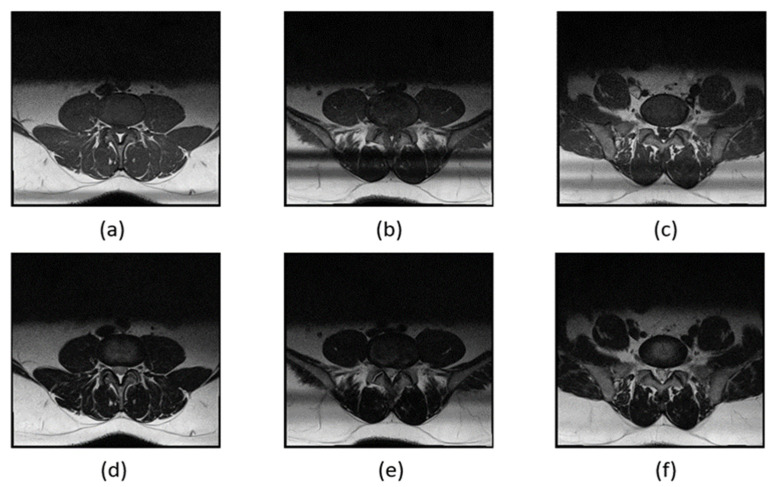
Spinal canal in different parts in T1–T2. From Figure 8, it can be observed that there are slight differences in pictures (a–f). (a) The facet joints of the spinal canal in the MRI images of L3 and L4 are more obvious, and the L5 site is connected to the S1 site; (b) therefore, the shape of the spinal canal in the MRI image will be more special. (c) T1 and T2 weighting also had a significant influence on the image. (d) T1 weighting will enhance the fat part in the MRI image; (e) T2 weighting will strengthen the watery part in the image, and thus the spinal canal part in the T1 weighted MRI image appears to be completely black, (f) whereas in T2-weighted MRI images, the spinal canal is lighter and some white nerves can be observed.
